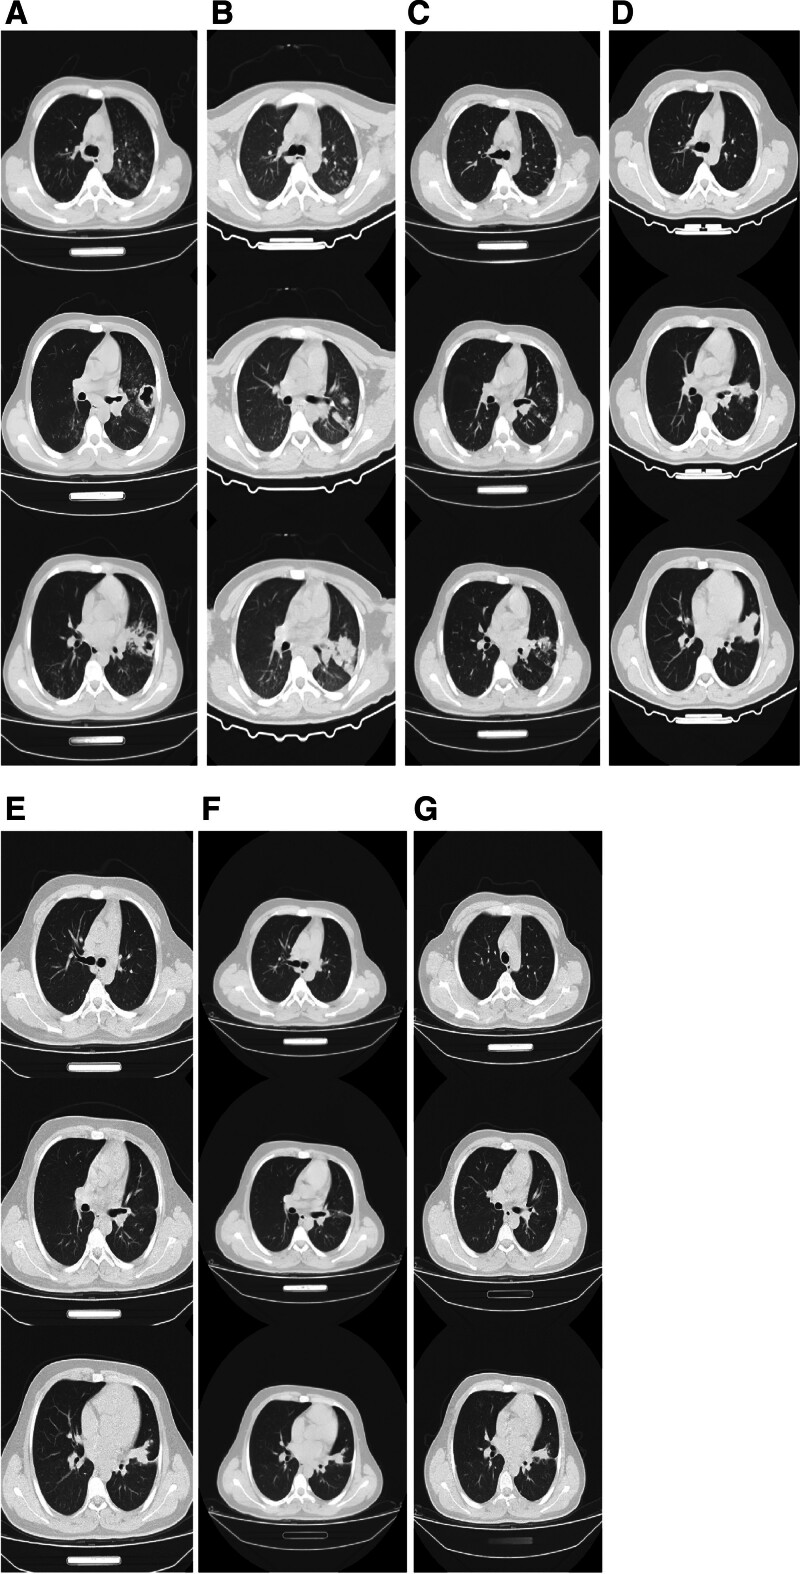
Progression of the patient’s chest CT images during treatment. The images were taken in (A) March 2023, (B) May 2023, (C) July 2023, (D) October 2023, (E) February 2024, (F) August 2024, and (G) December 2024.
